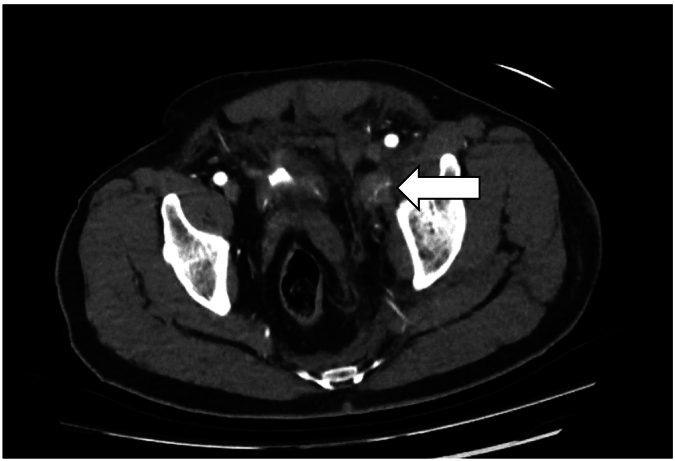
Axial CT Pelvis with deflated bladder and enhancing left hematoma (arrow).
